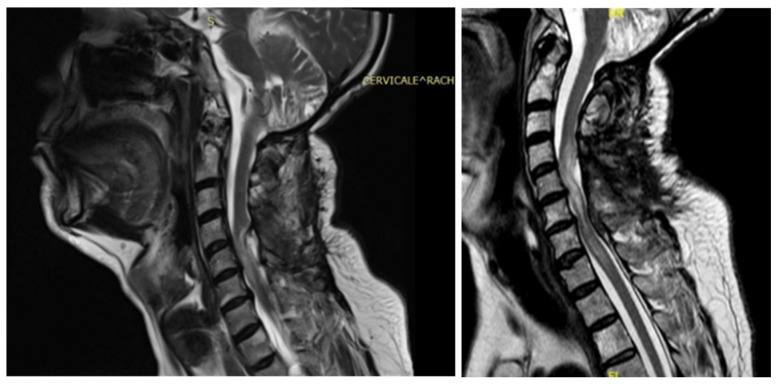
Case 2: pre-operative MRI (left) and post-operative MRI (right).
